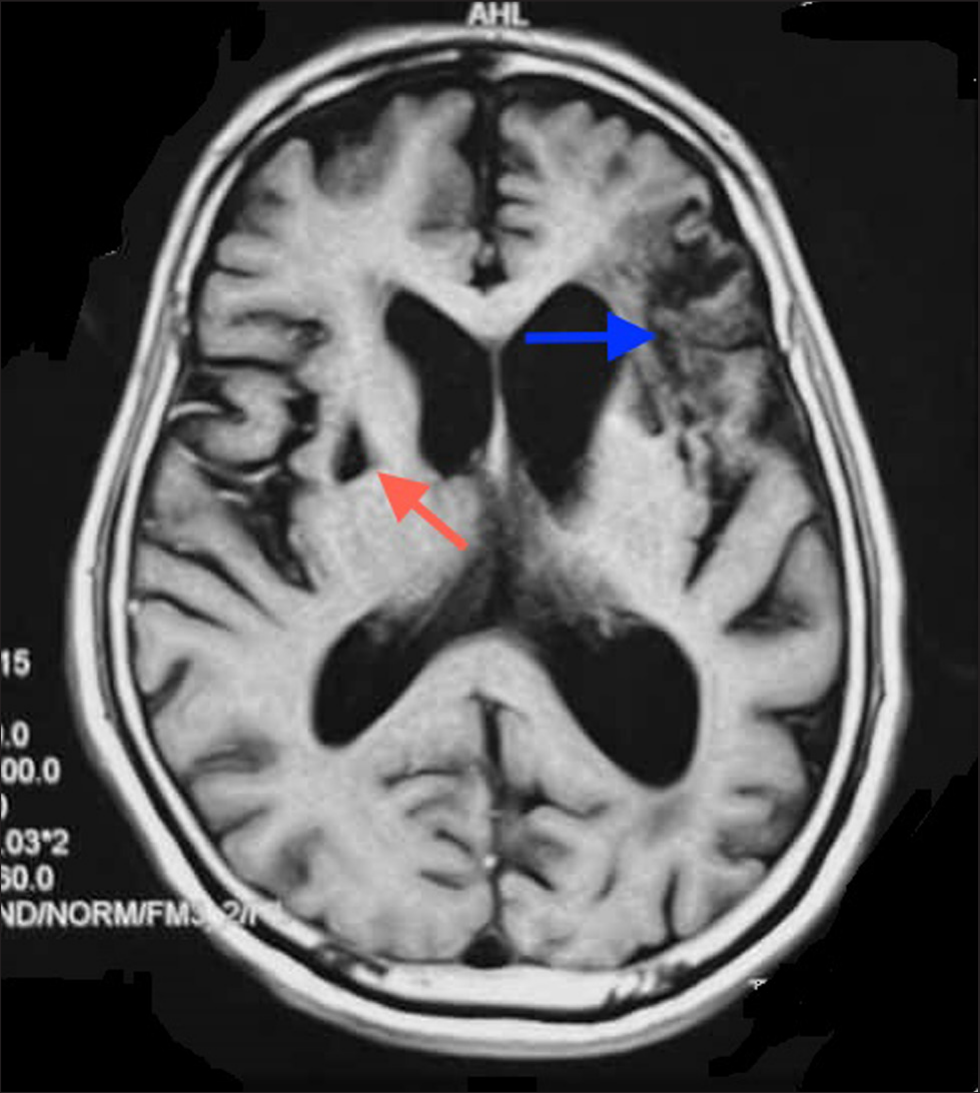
Axial T1 weighted MR image shows irregular signal characteristics in left lateral frontal cortex and white matter extending to operculum, precentral gyrus, sylvian cortex (blue arrow) as well as gliosis in right putamen and head of caudate nucleus (red arrow).
